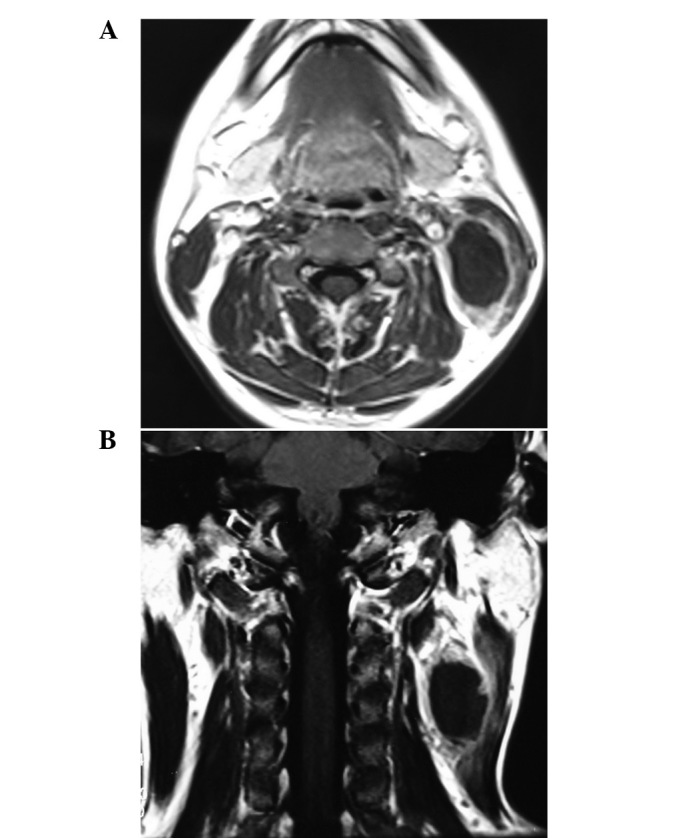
Type II BCC with infection. (A) A left, ill-defined cystic mass presented with enhanced irregular thickening of the wall on contrast-enhanced T1WI. (B) A small protruding lumen was observed at the lateral wall in coronal planes. BCC, branchial cleft cyst; T1WI, T1-weighted image.
